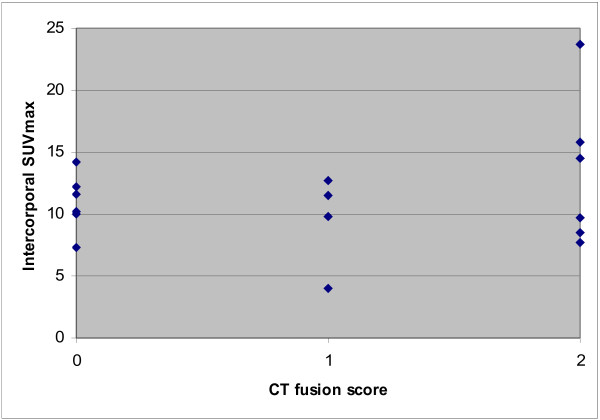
^18^F-fluoride activity in vertebral fusion. Intervertebral SUVmax values with corresponding fusion scores on CT.
